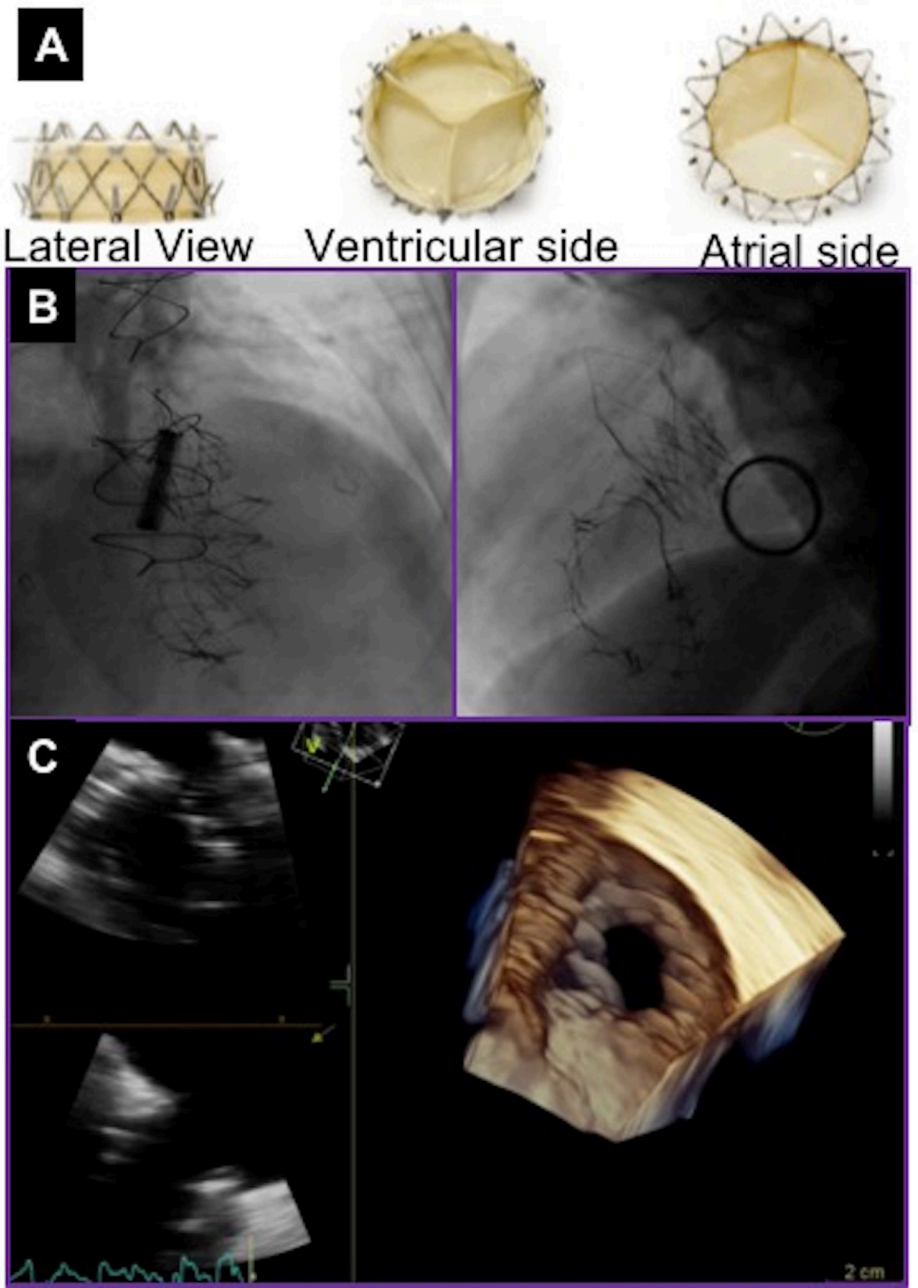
NaviGate valve and final result after valve deployment. (A) NaviGate Valve Profile. (B) Fluoroscopic images of NaviGate deployed in tricuspid annulus with relative relations with mitral valve (previous mitral valve replacement) and aortic valve (previous transcatheter aortic valve implantation). (C) Echocardiographic images showing good expansion and stable position of the NaviGate Valve.
